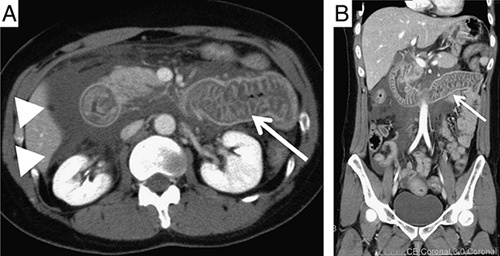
A and B, Abdominal computed tomography images during an attack of hereditary angioedema revealed intestinal edema (arrow) and ascites (arrowheads). Reproduced without changes under Creative Commons Attribution-NonCommercial 4.0 International license from Hirose et al.^60^
